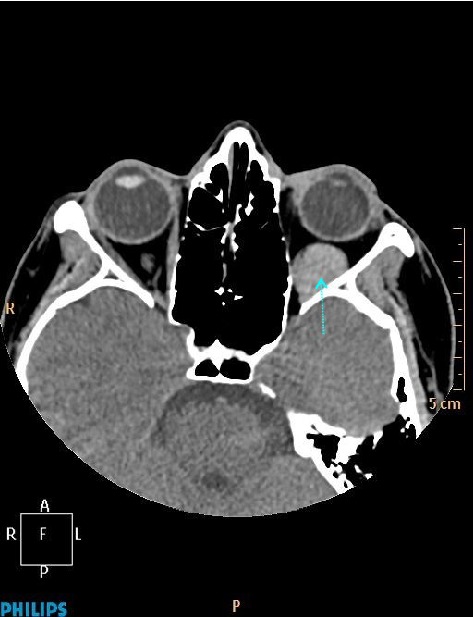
Axial noncontrast CT image showing a hyperdense retrobulbar mass in left orbit (arrow).
